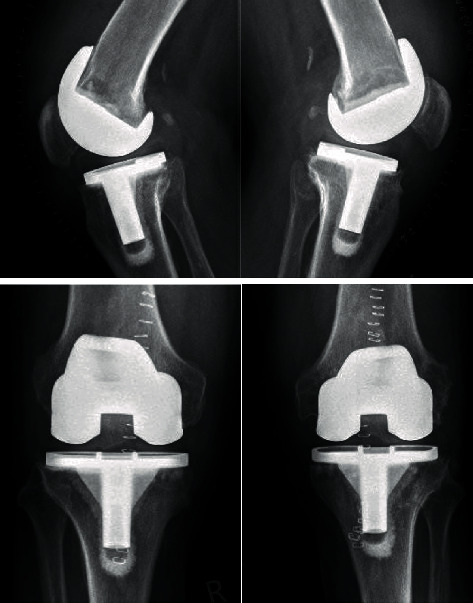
Radiographs in 2 planes directly after the implant-retaining procedure on both sides10 years after TKAs: no hint for loosening and clip suture.
